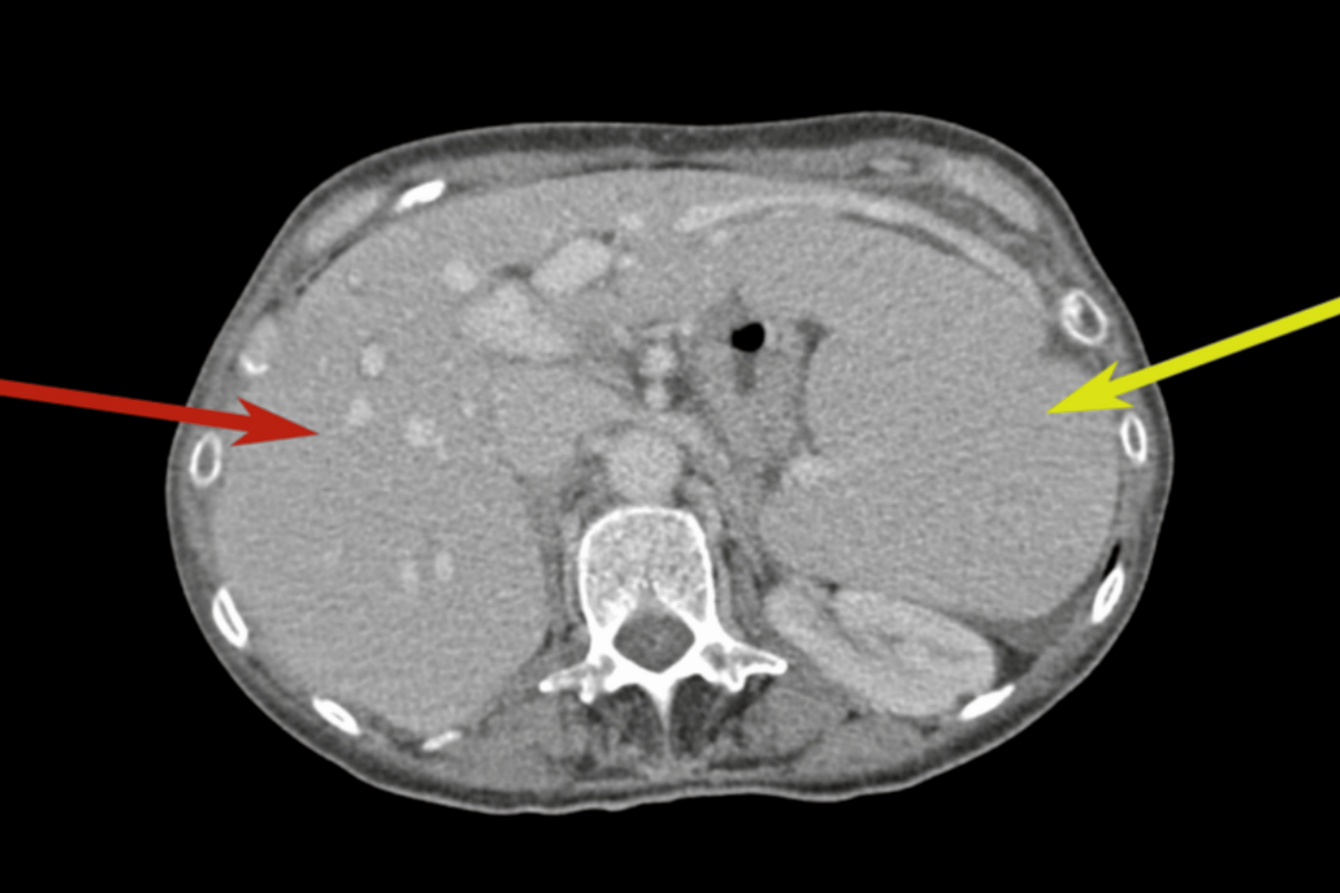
CT scan demonstrating hepatosplenomegaly The red arrow indicates hepatomegaly and the yellow arrow highlights splenomegaly.
